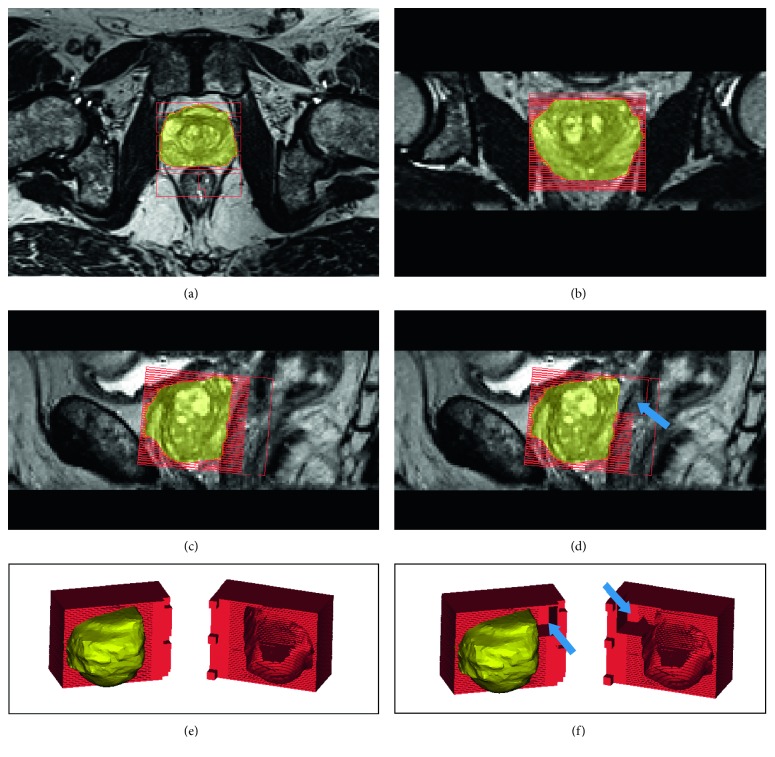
Visualization of the slicer overlaid to the MRI of the prostate imaged in three different planes and after 3D modeling. This procedure enables simple checking for correct positioning and alignment of the slicer with respect to the image acquisition axes. (a, b) Axial and coronal plane. (c) Sagittal plane with slicer without the seminal vesicles space. (d) Sagittal plane, a model that takes also into account a space (arrows) for the seminal vesicles after prostatectomy. (e, f) The 3D view of slicers with the prostate, without and with (arrows) space for seminal vesicles.
